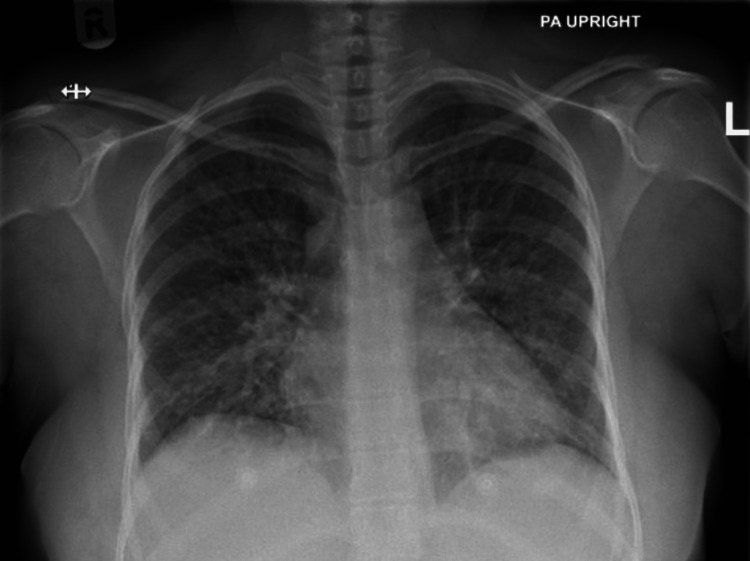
Chest X-ray showing widespread areas of airspace opacities in the bilateral lung zones
